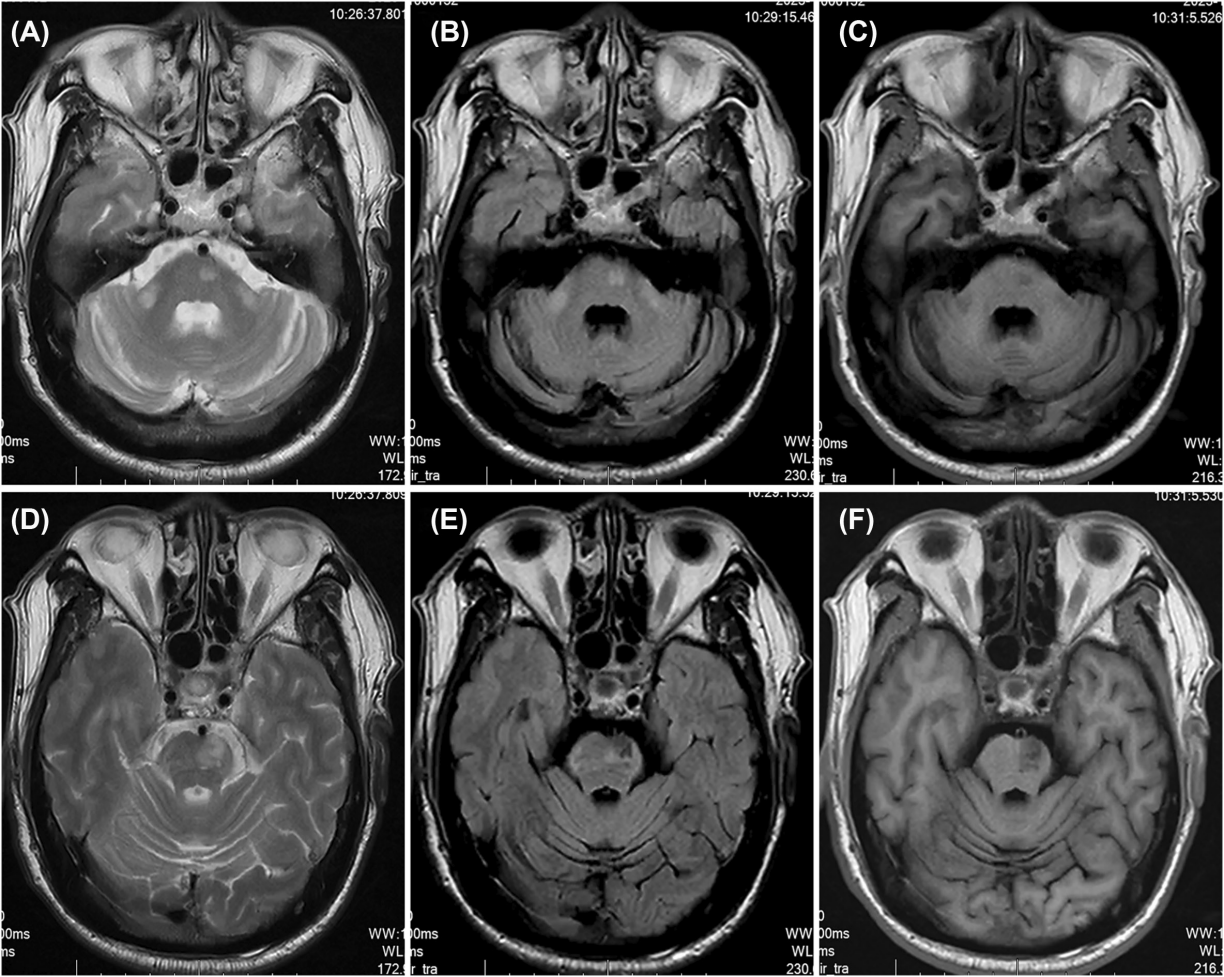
MRI showed bilateral symmetrical wallerian degeneration in middle cerebellar peduncles (patient 3). Symmetrically high T2‐weighted (A) and fluid‐attenuated inversion recovery imaging (B) signal intensities, with low T1‐weighted (C) signal in the bilateral peduncles. Low T1W (D) signal intensities in the left pons, with high T2W (E) and FLAIR (F) signal intensities.
